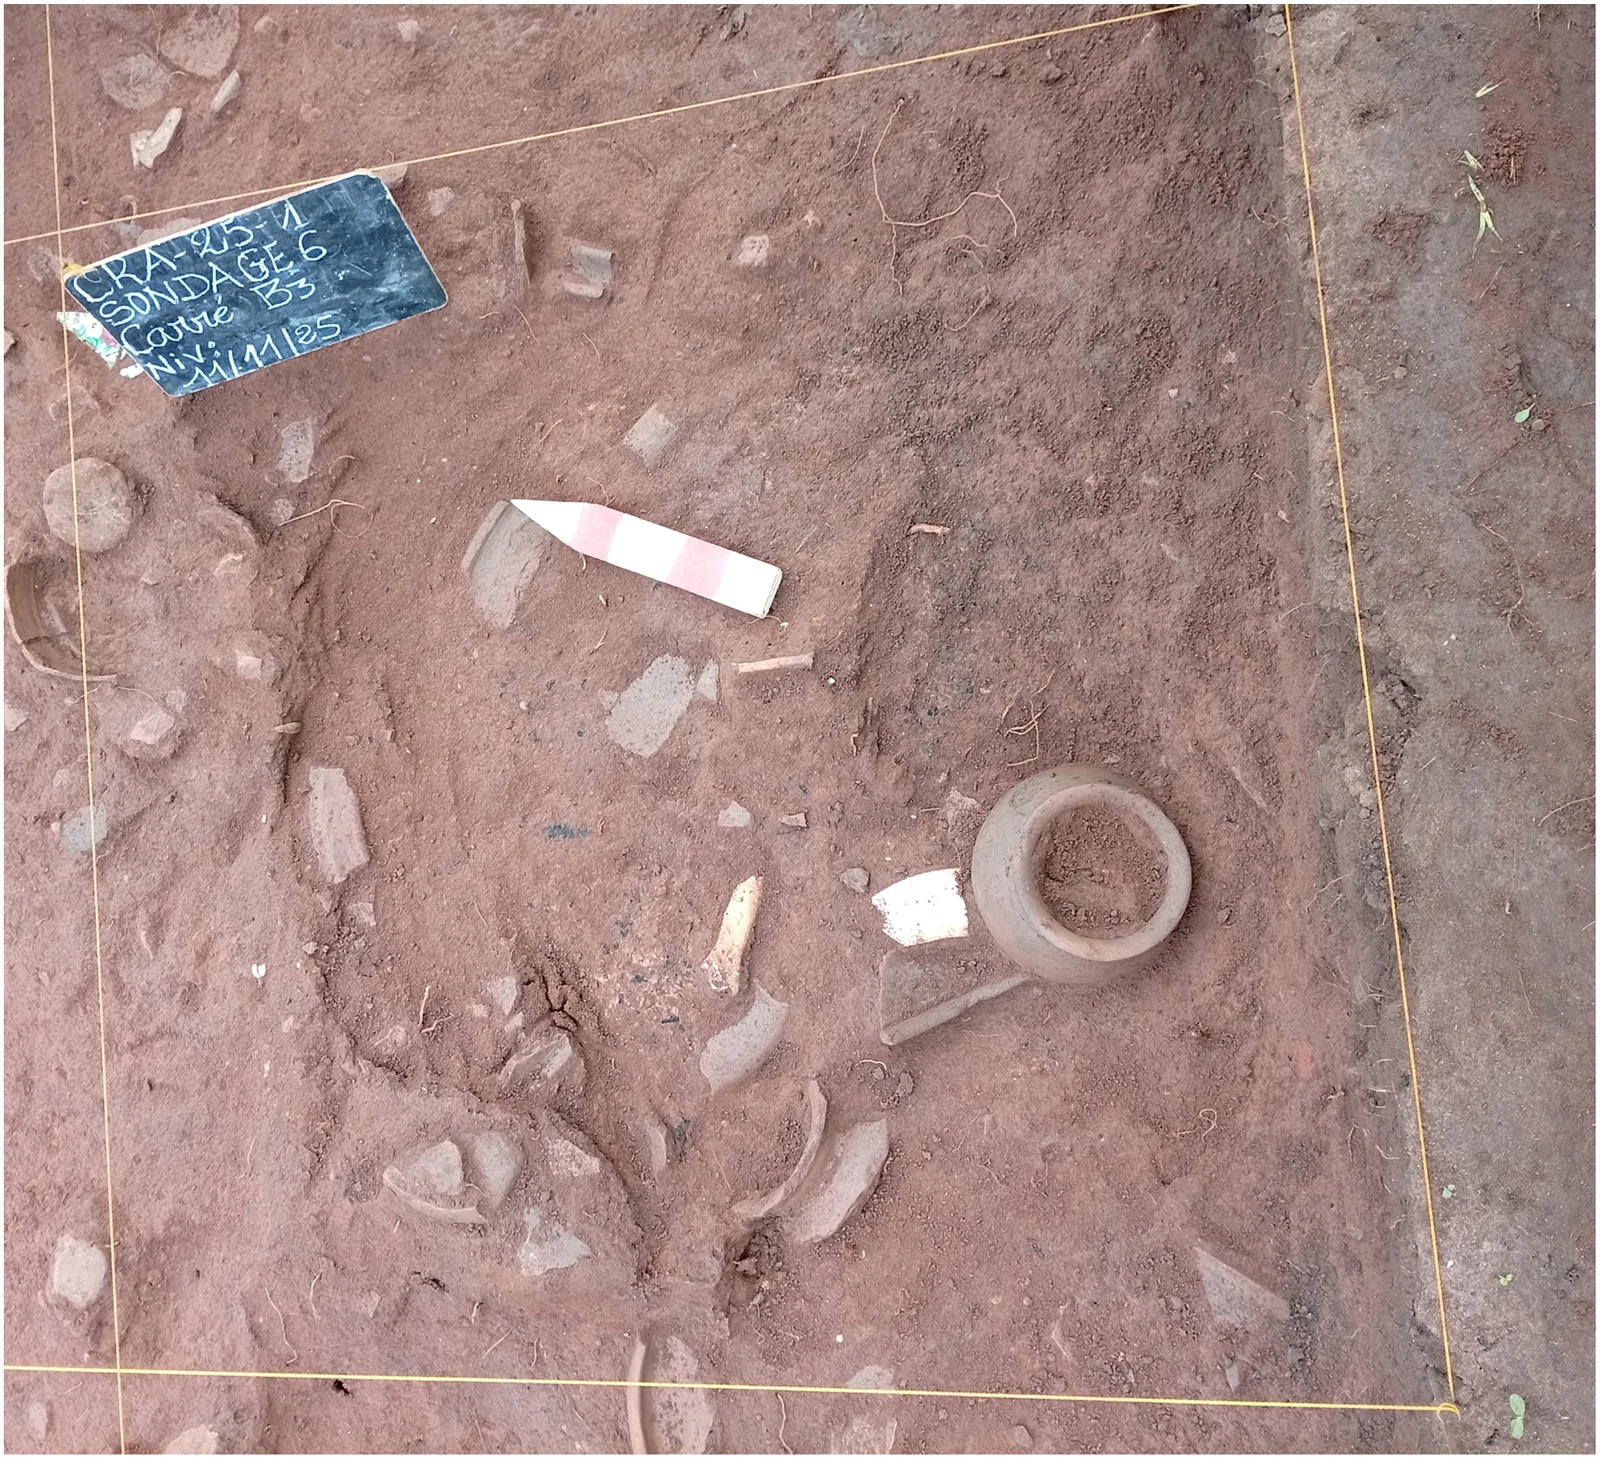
CT-scan examination of sacred Vodun objects (Benin): In-situ aspect of the ceramics discovered in the archaeological excavations at Abomey.
